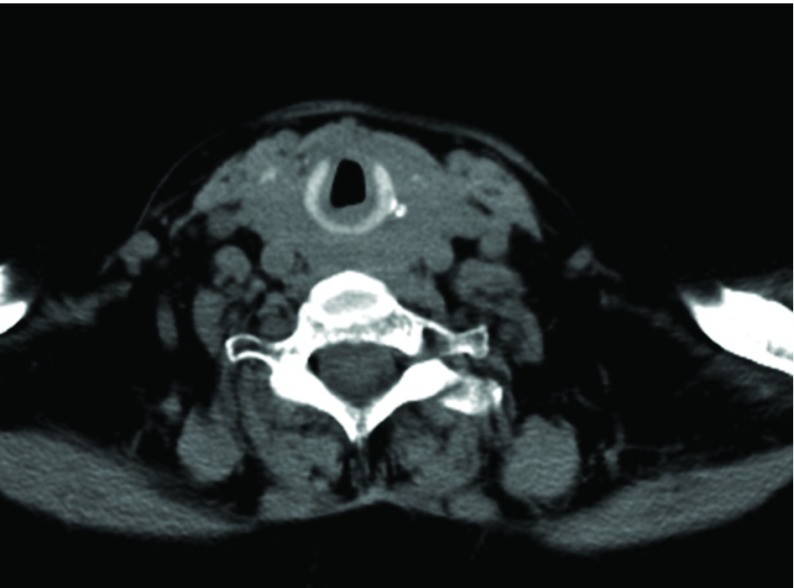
The CT Scan Showing the Absence of Significant Tracheal Compression
